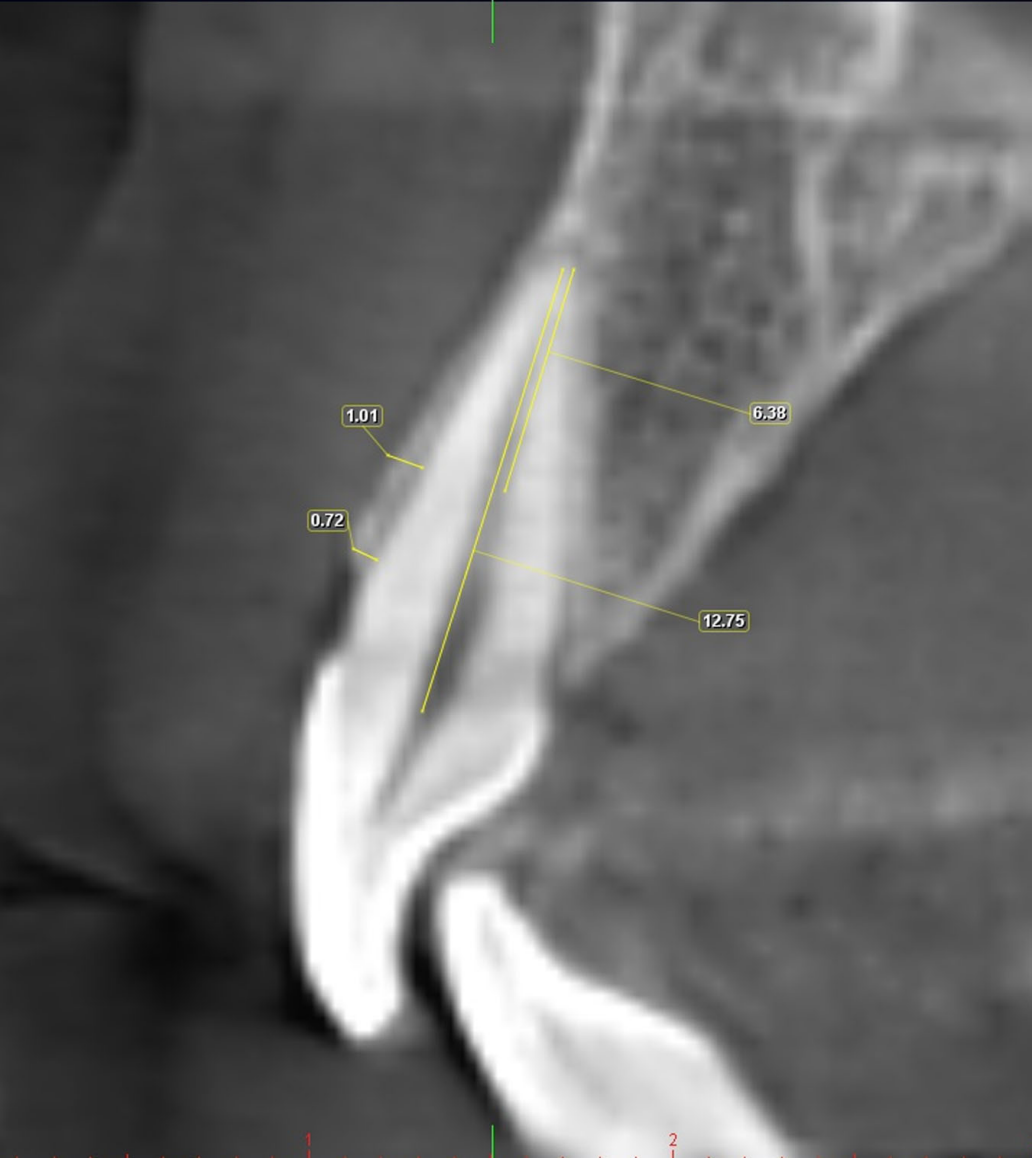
Evaluation of CBCT images from sagittal view. Maximum root length was measured from the apical point and the CEJ. Half of the measured length was determined as the midroot. Buccal bone thickness was measured at two points (crestal and midpoint).
